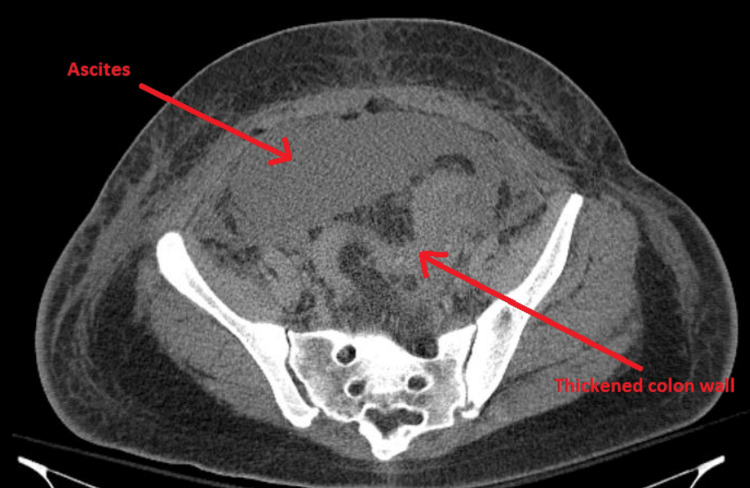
CT of abdomen/pelvis. Arrows represent thickened colon wall and ascites.
